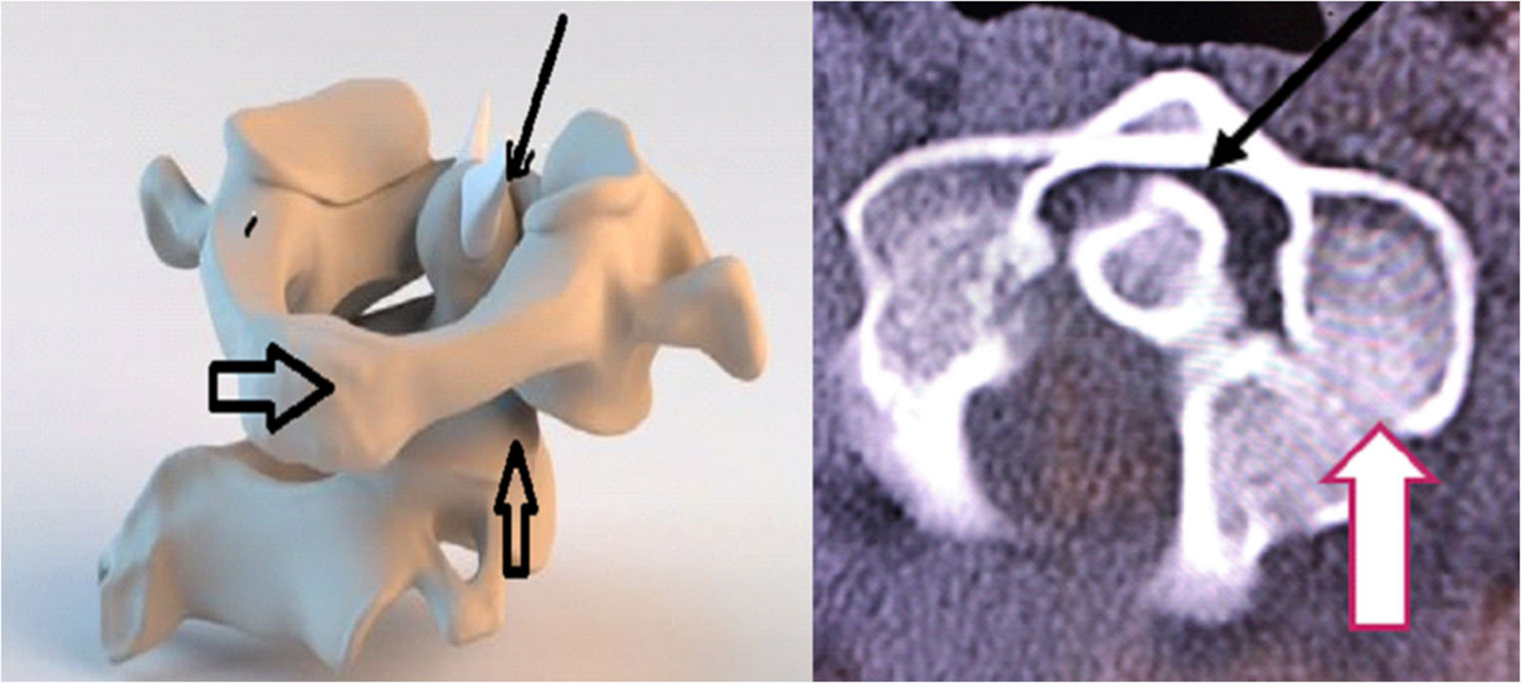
The model in the left image demonstrates the rotary subluxation (AAI Fielding type 1) that occurs with the hereditary connective tissue disorders. With the rotation of the C1 ring (large black arrow), the atlanto-dental interval (small solid arrow) remains normal (< 3 mm), but the facet joint (vertical arrow) is subluxed with more than 80% loss of facet overlap, and the angular displacement between C1 and C2 exceeds the pathological threshold of 41 degrees. On the right, the cervical spine CT (axial view through the interface of C1 and C2) shows the loss of facet overlap (vertical white arrow), but normal atlanto-dental interval (black arrow), representing a rotary subluxation, Fielding Type 1
